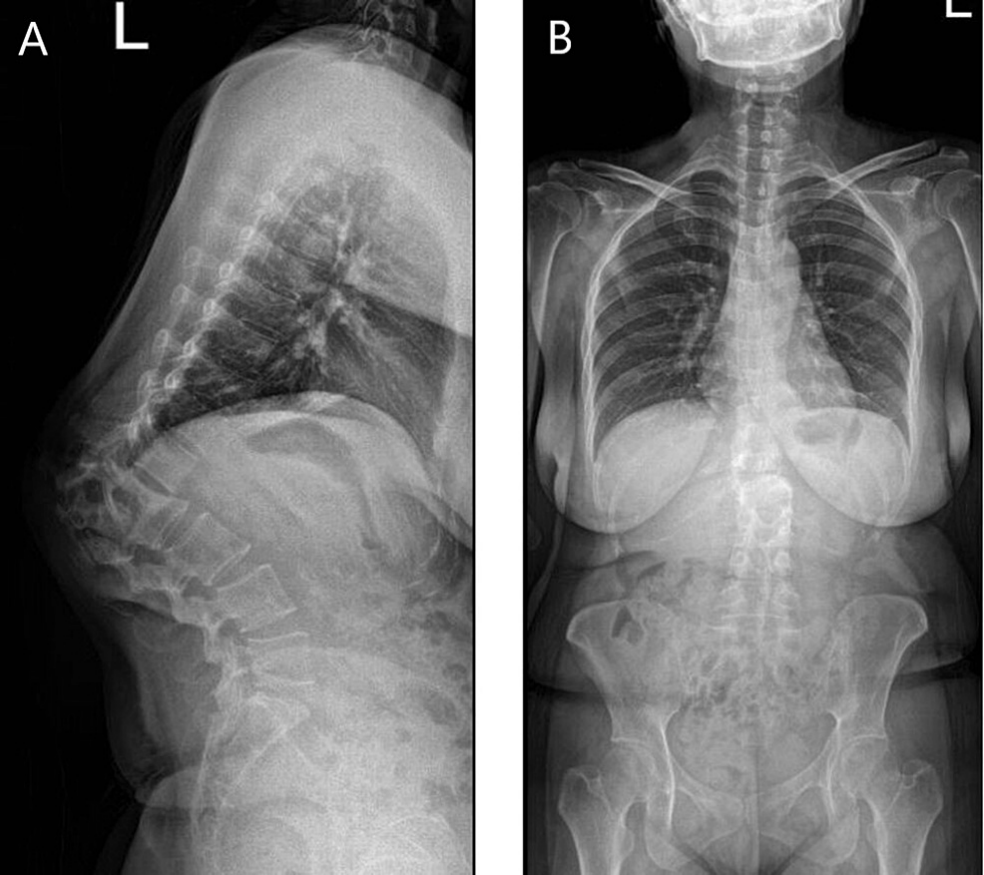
Preoperative lateral (A) and anteroposterior (B) whole-spine radiographs of the patient's severe kyphosis.
